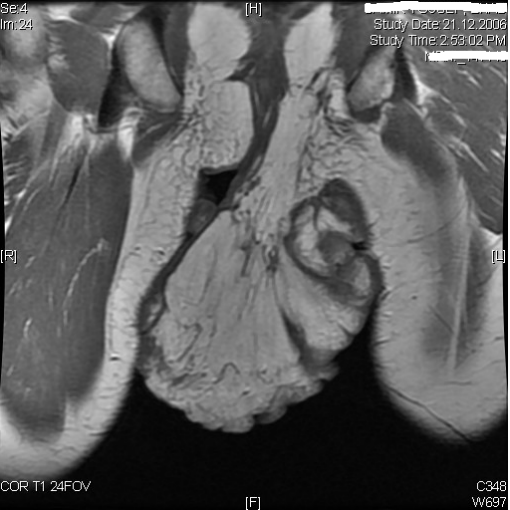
T1-MRI axial section showing fatty signal intensity with evidence of solid component that showed moderate enhancement suggestive of liposarcoma.
